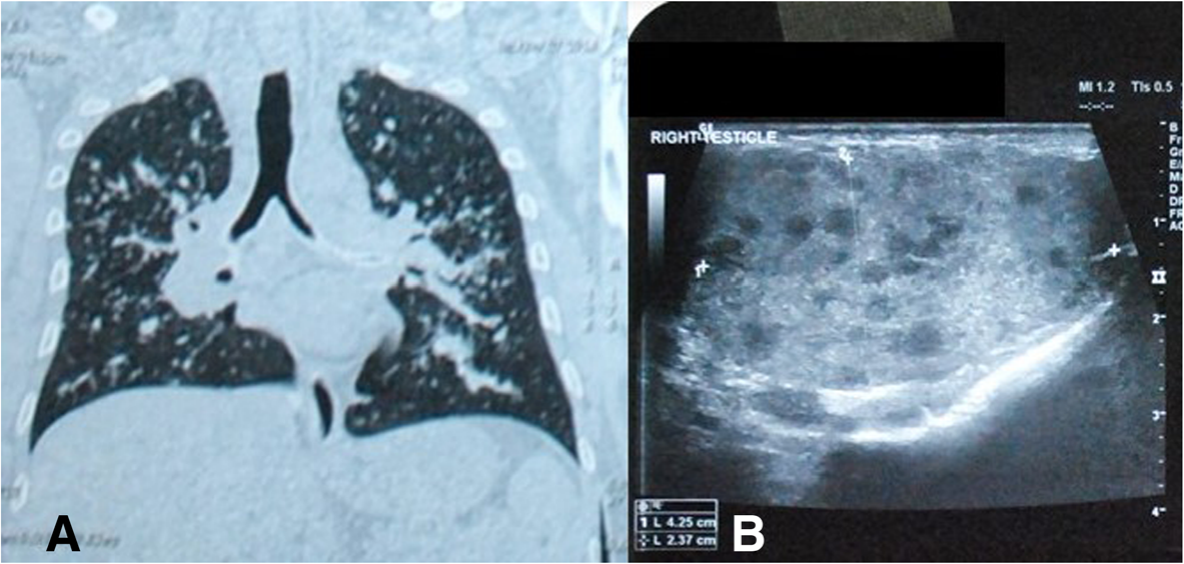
a. HRCT chest showing multiple areas of nodular consolidation and nodules along the fissures, mediastinal and hilar lymphadenopathy, and bilateral basal pleural thickening b. Ultrasound Doppler study of scrotum showing mild epididymis echotexture with small cysts
